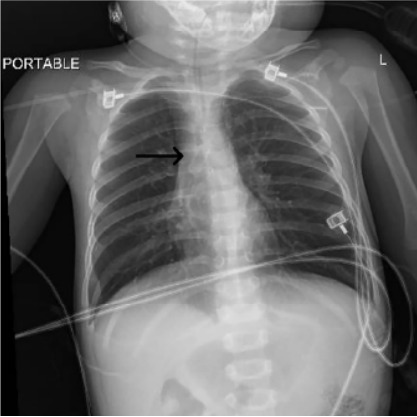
Chest radiograph of a 16-month male with foreign body (arrow) after endotracheal intubation.
